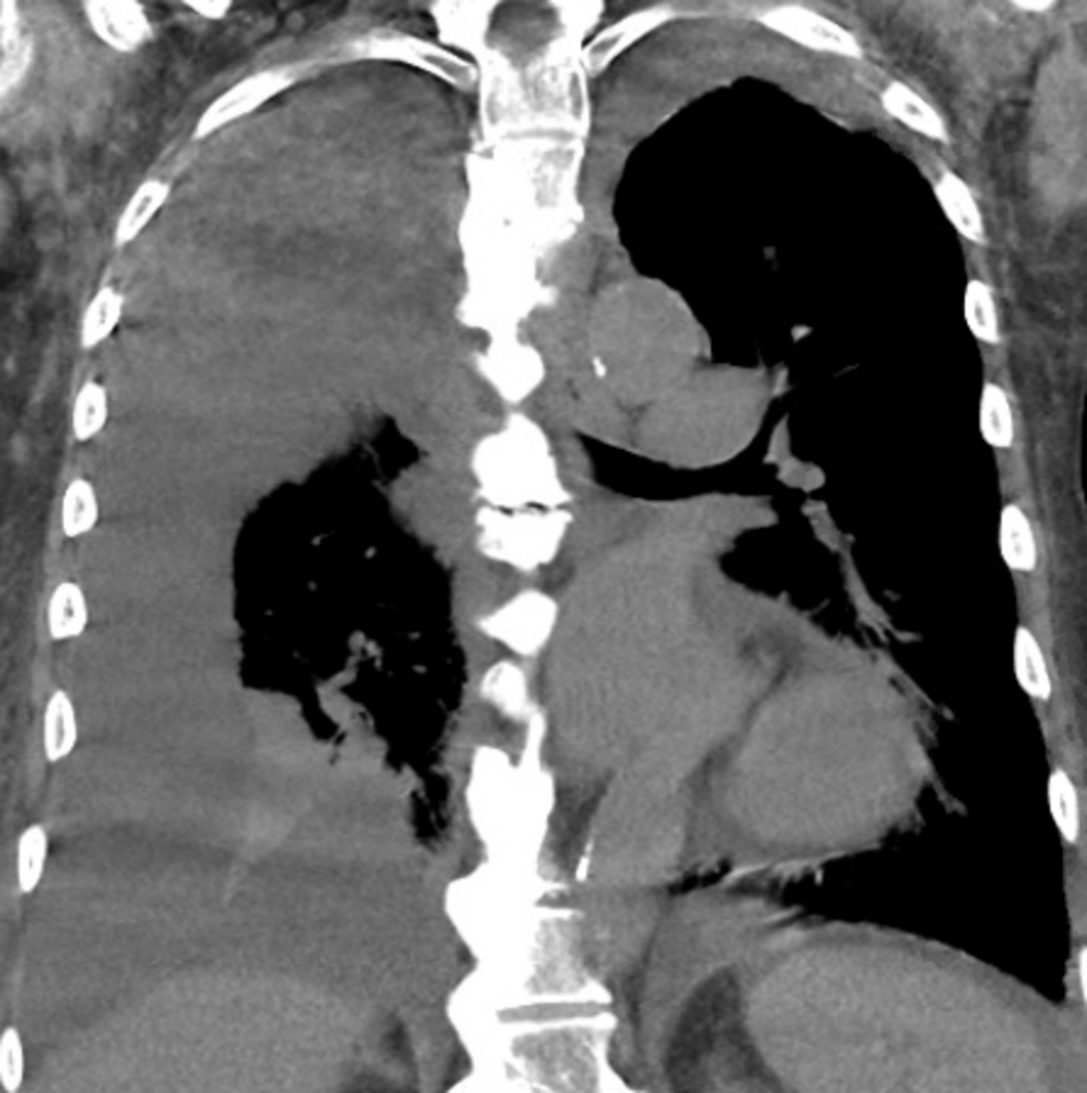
Computed tomography of the chest showed right-sided pleural effusion
